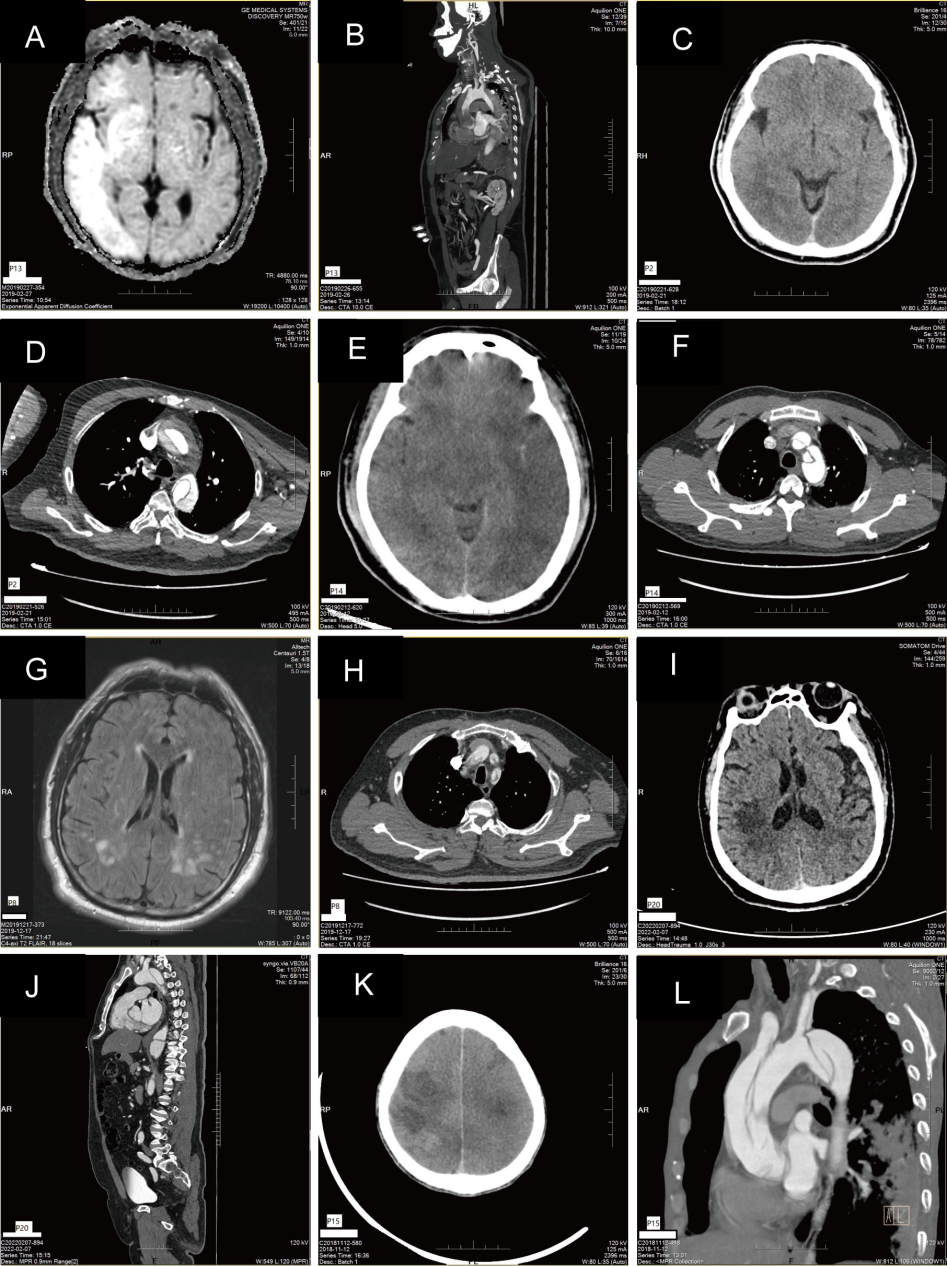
Imaging of aortic dissection and cerebral infarction. (A) MRI
